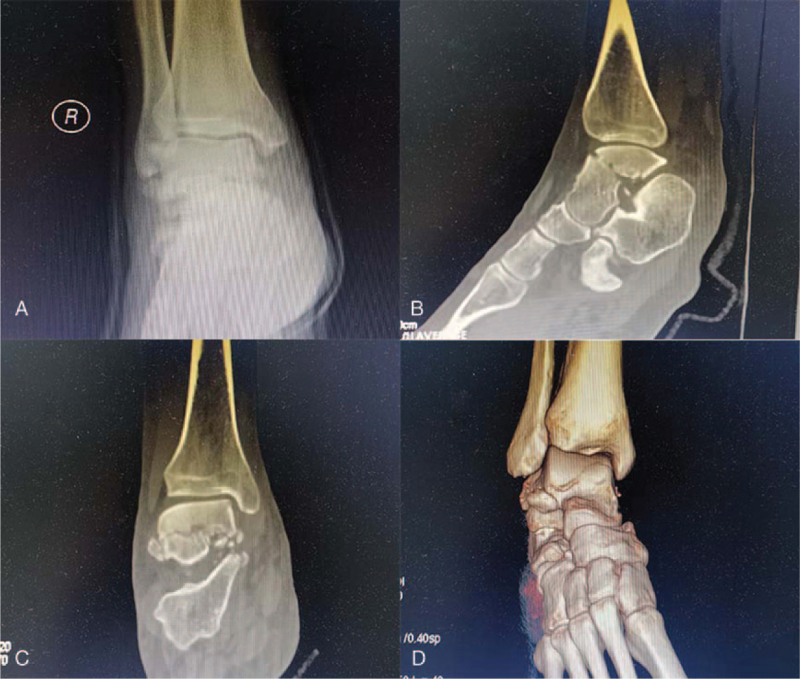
X-ray and CT examination showing right talus fracture. (A) Anterior-posterior view. (B) Sagittal image of CT.(C) Coronal image of CT. (D) 3-Dimensional reconstruction of CT.
